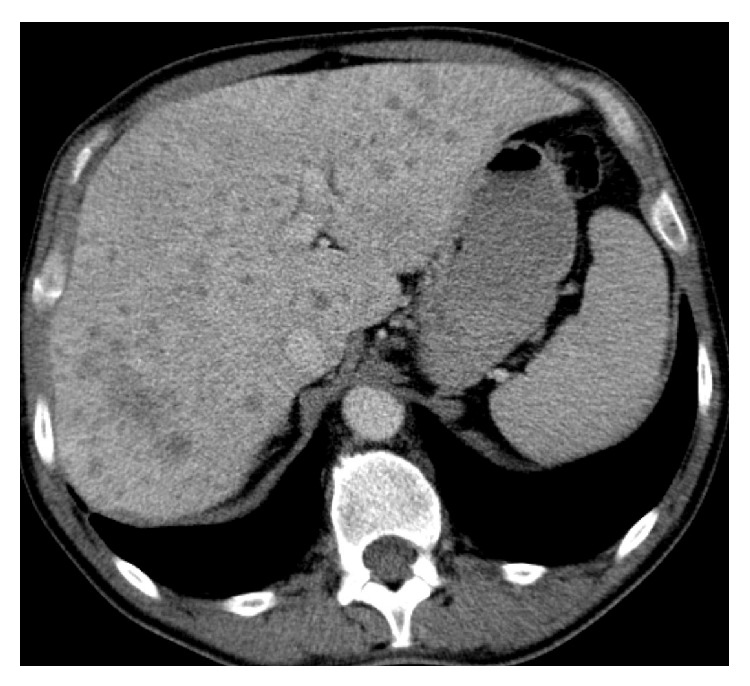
Axial contrast-enhanced abdominal CT: the patient presented increased liver dimension (hepatomegaly) in a biopsy proven liver acute involvement of LCH. Multiple hypodense nodules are identified, some of which confluent. No bile duct dilatation is present.
